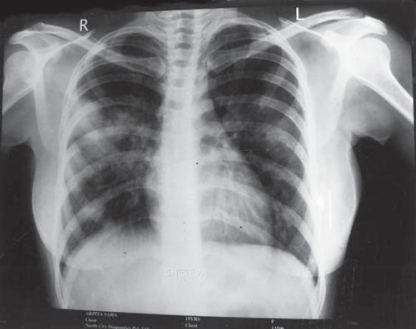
Initial chest radiograph showing bilateral pulmonary unevenly distributed nodular opacities with coalescence of some pulmonary nodules.
